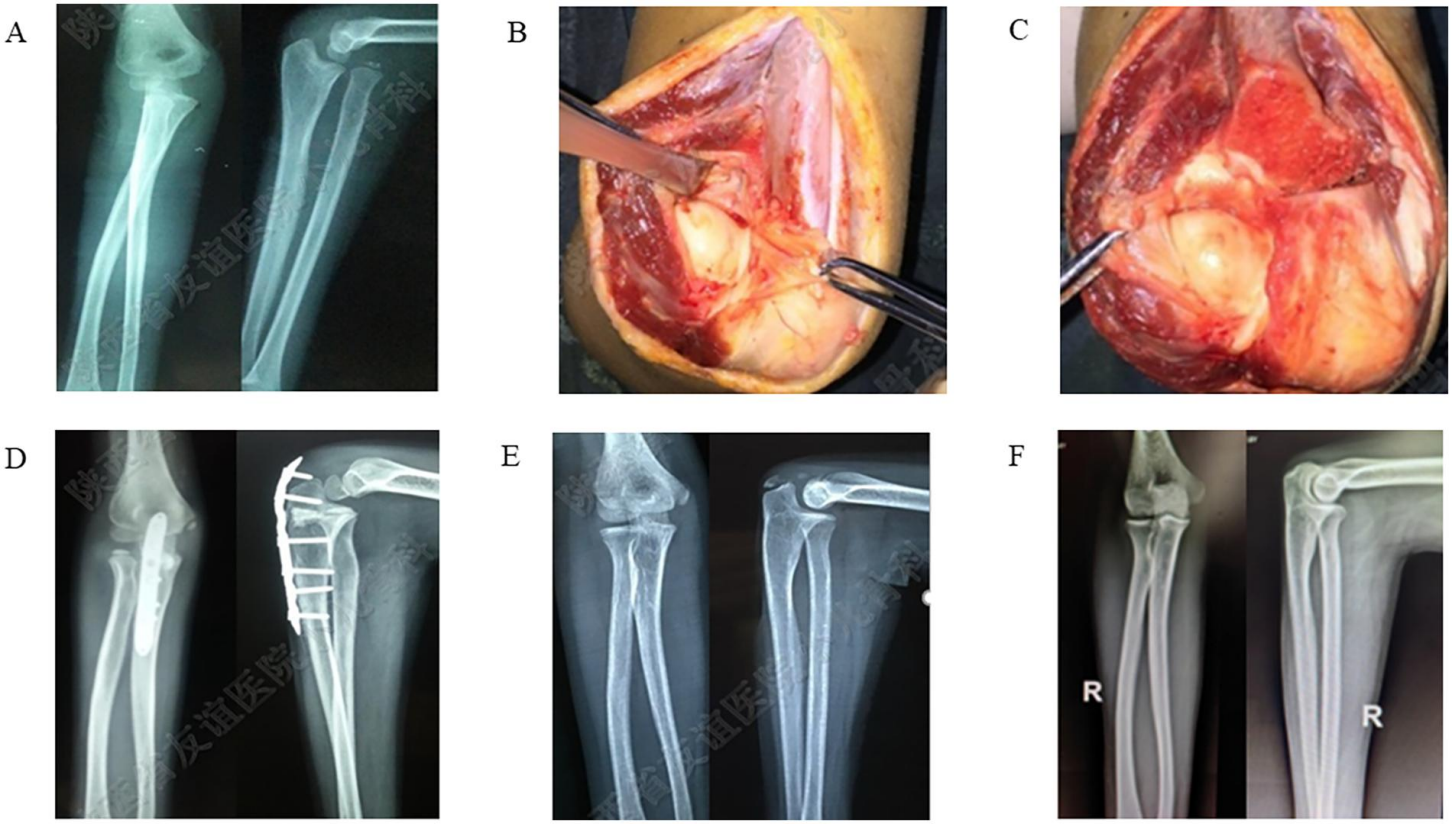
A 7-year-old male patient with a congenitally dislocated radius head on the right side. (A) Preoperative elbow x-ray shows anterior dislocation of the radial head; (B,C) Intraoperative photographs reveal proximal overlap of the ulna and radius, cubitus valgus deformity, and intact radioulnar joint. (D) Immediate postoperative DR demonstrates a well-positioned radial head, with normal radioulnar and humeroradial relationships. (E,F) DR images at 3 years and 10 years postoperatively show no dislocation of the radial head, normal elbow joint relationships.
